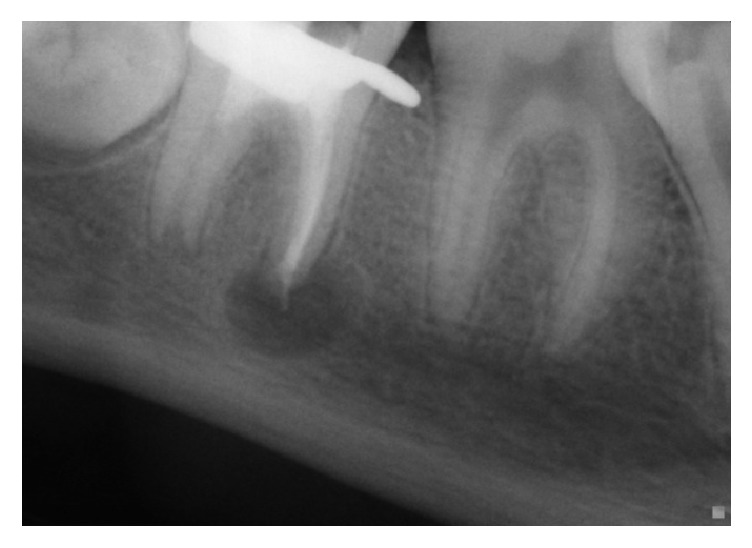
Radiograph 7 days after endodontic therapy.
